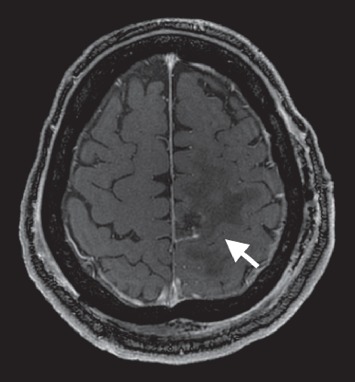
Postresection, T1 postgadolinium MRI brain with axial view. This reveals postsurgical changes after a gross total resection of the left frontoparietal mass with residual edema in the tumor bed.
